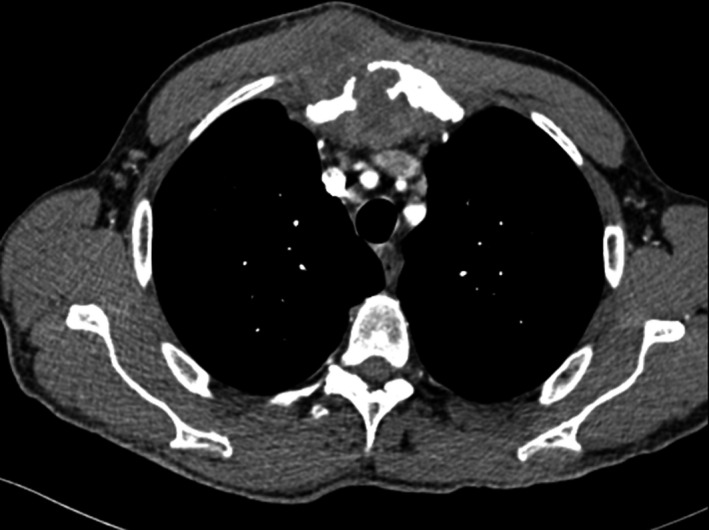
Second CT of the chest showing local progression
